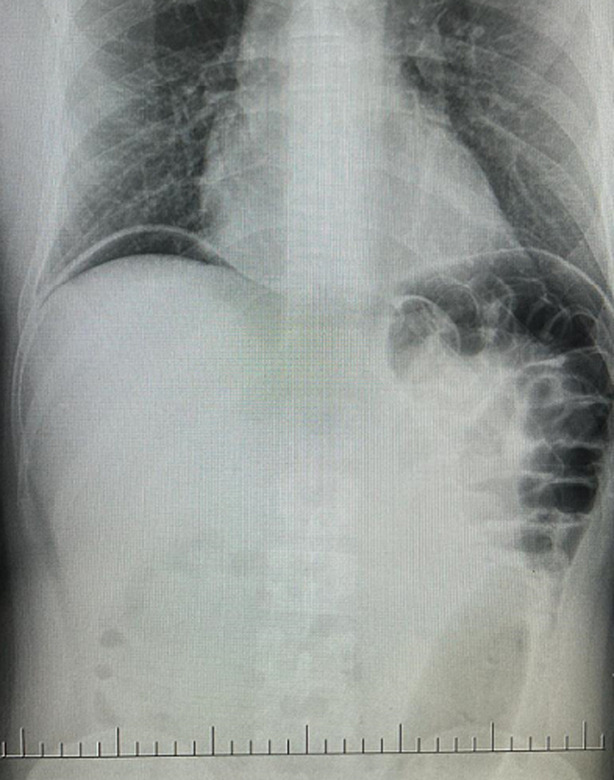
plain abdominal X-rays showing free air-stripes under both hemidiaphragm and in the peripherals
